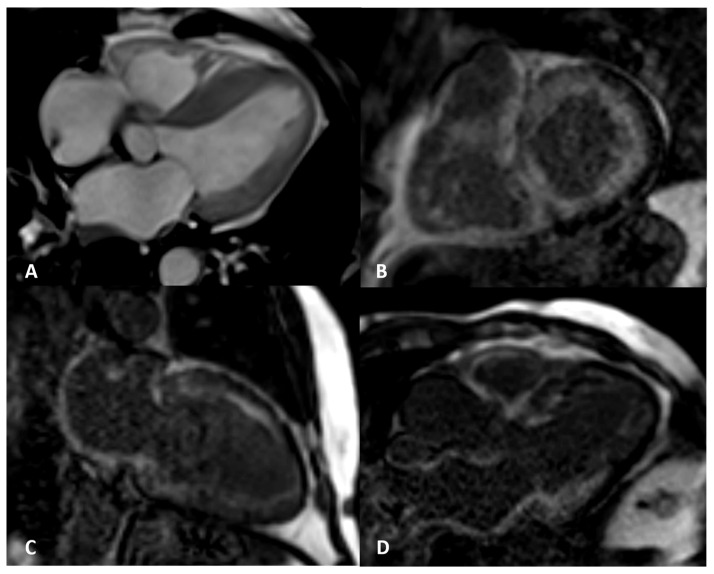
AL amyloidosis confirmed by endomyocardial biopsy. An elderly patient with NYHA III heart failure and 42% LVEF. Four-chamber balanced-steady state free precession (b-SSFP) MRI (A) shows symmetric thickening of the left ventricle with the septum maximally measuring 25 mm. Circumferential subendocardial LV LGE in two-chamber short (B) and long axes (C) and three-chamber b-SSFP (D); QALE score 12.
